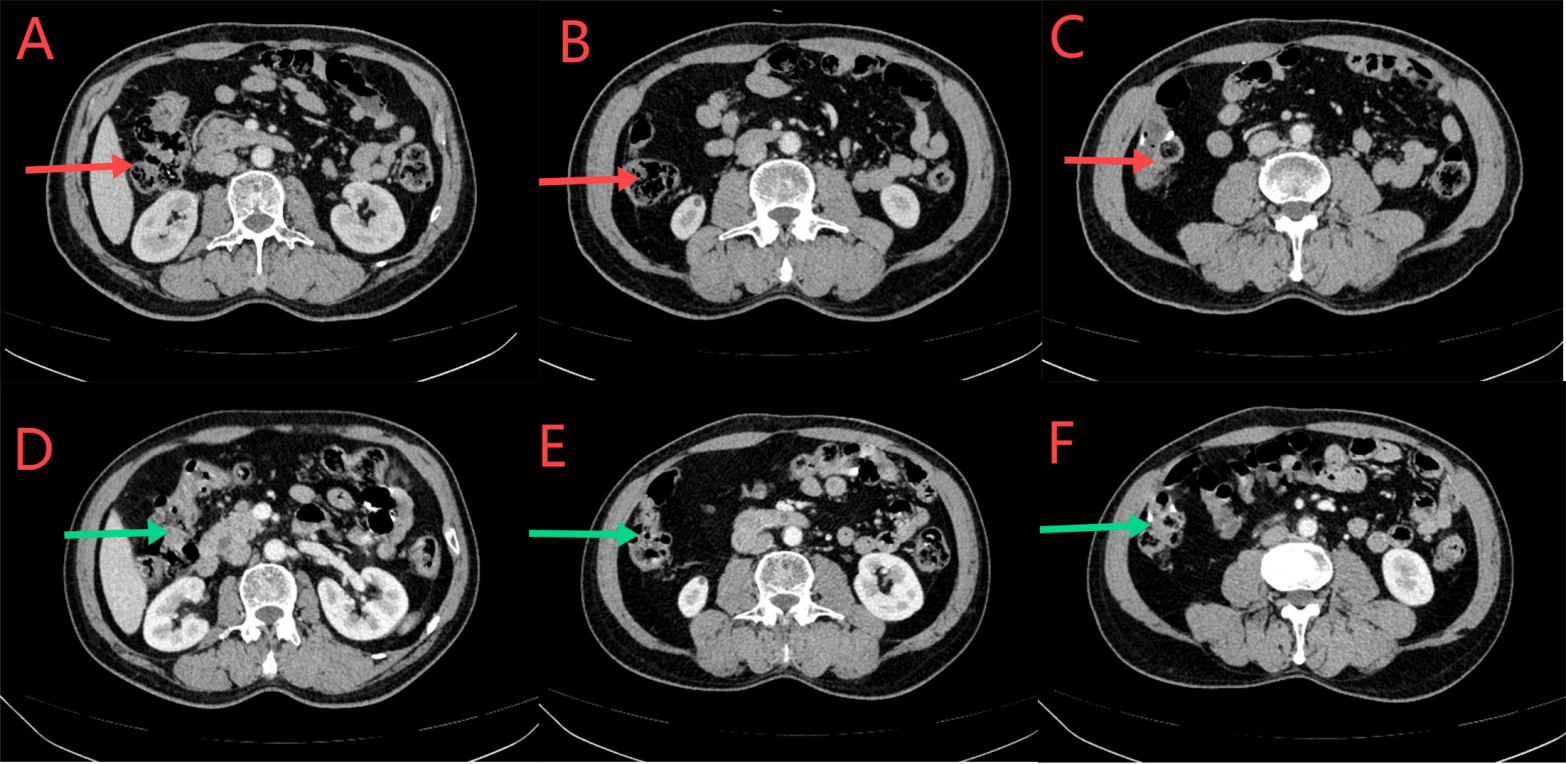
(A–C) CT scan conducted on March 12, 2024. The red arrows indicate normal local growth of the anastomotic and distal intestinal wall, with small lymph nodes visible in the surrounding fat space. According to the clinical data, there was no significant change compared to the CT scan on December 26, 2023. (D–F) CT scan conducted on June 6, 2024. Green arrows indicate local thickening of the anastomotic and distal intestinal walls; Small lymph nodes in the surrounding fat space are visible. There was no significant change compared with the CT scan on March 12, 2024.
